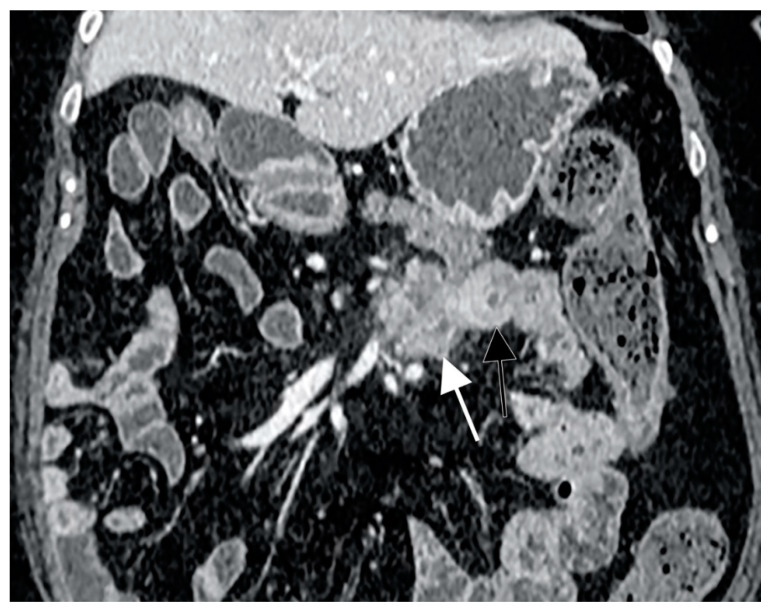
CT scan representation of small bowel carcinoma (SBC). White and black arrows show heterogeneous attenuation and moderate enhancement after contrast medium injection.
